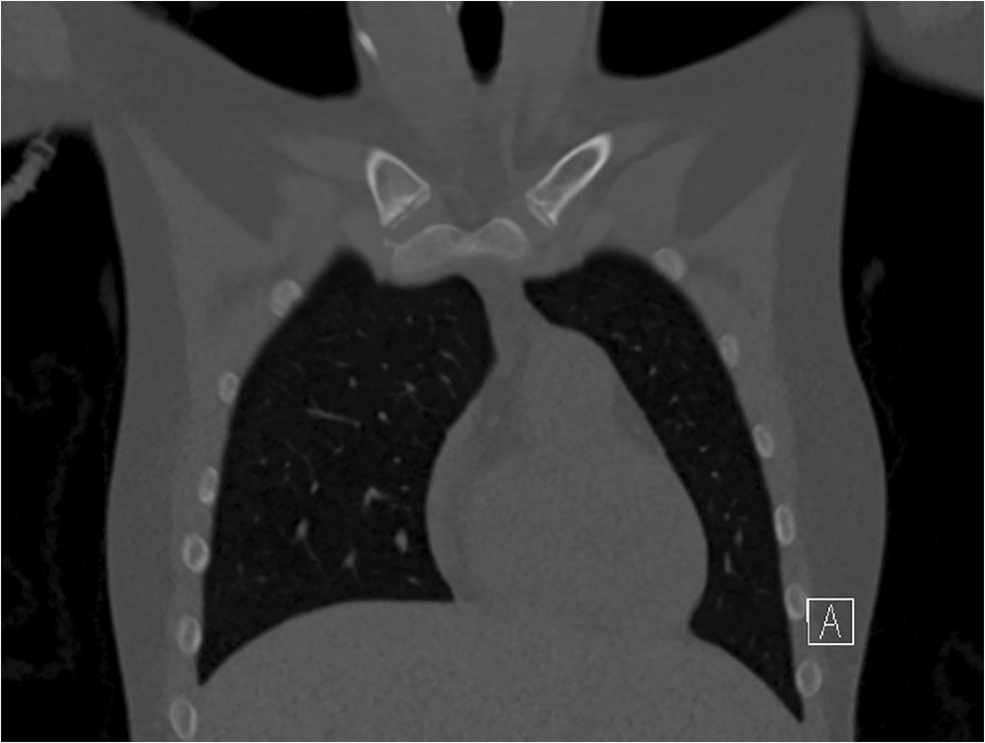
Coronal view of a chest CT using a low-dose imaging protocol (Sn100/reference 96 mAs; effective dose: 0.4 mSv). The ossification stage was classified as 2c
